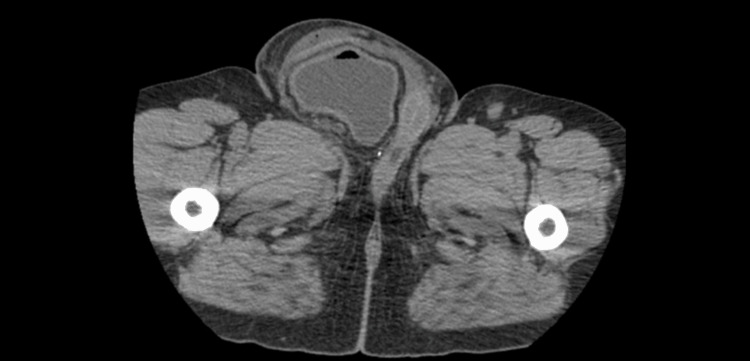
Axial view on CT demonstrating large right groin hernia containing bladder and fat with severe scrotal wall edema.
